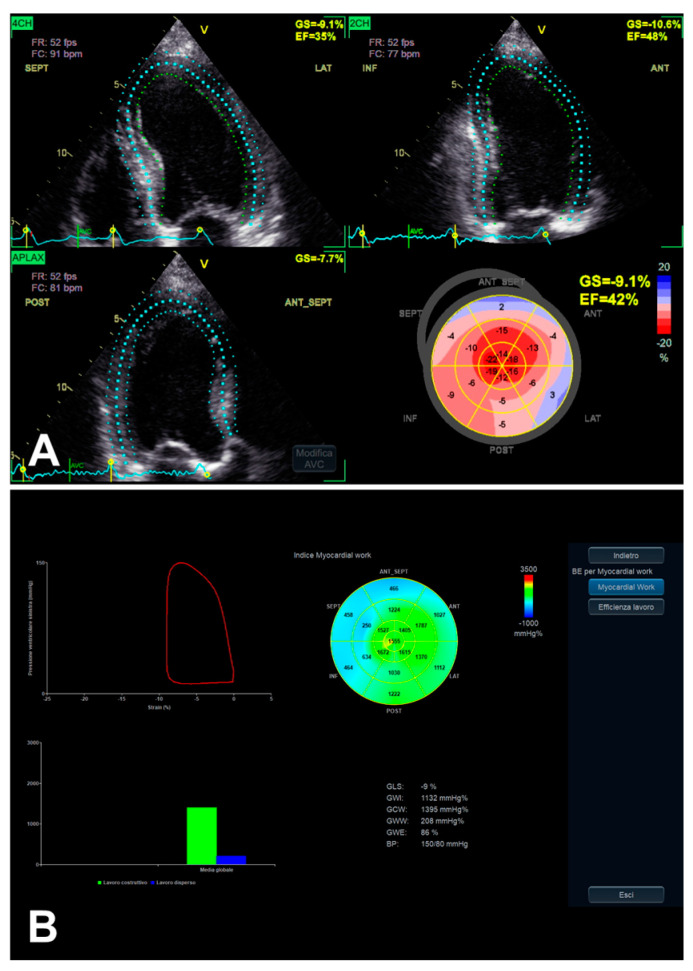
An example of LV function evaluation by LV GLS, in the presence of SAS and LV dysfunction, assessed from 2D apical four-chamber, three-chamber, and two-chamber views. When overt LV dysfunction occurs, the mentioned typical gradient between basal and apical segments in decreasing longitudinal function (initially in the presence of preserved LV EF) may finally develop an “apical sparing-like” picture (see “bull’s eye” plot in (A) and the corresponding MW analysis in (B)).
